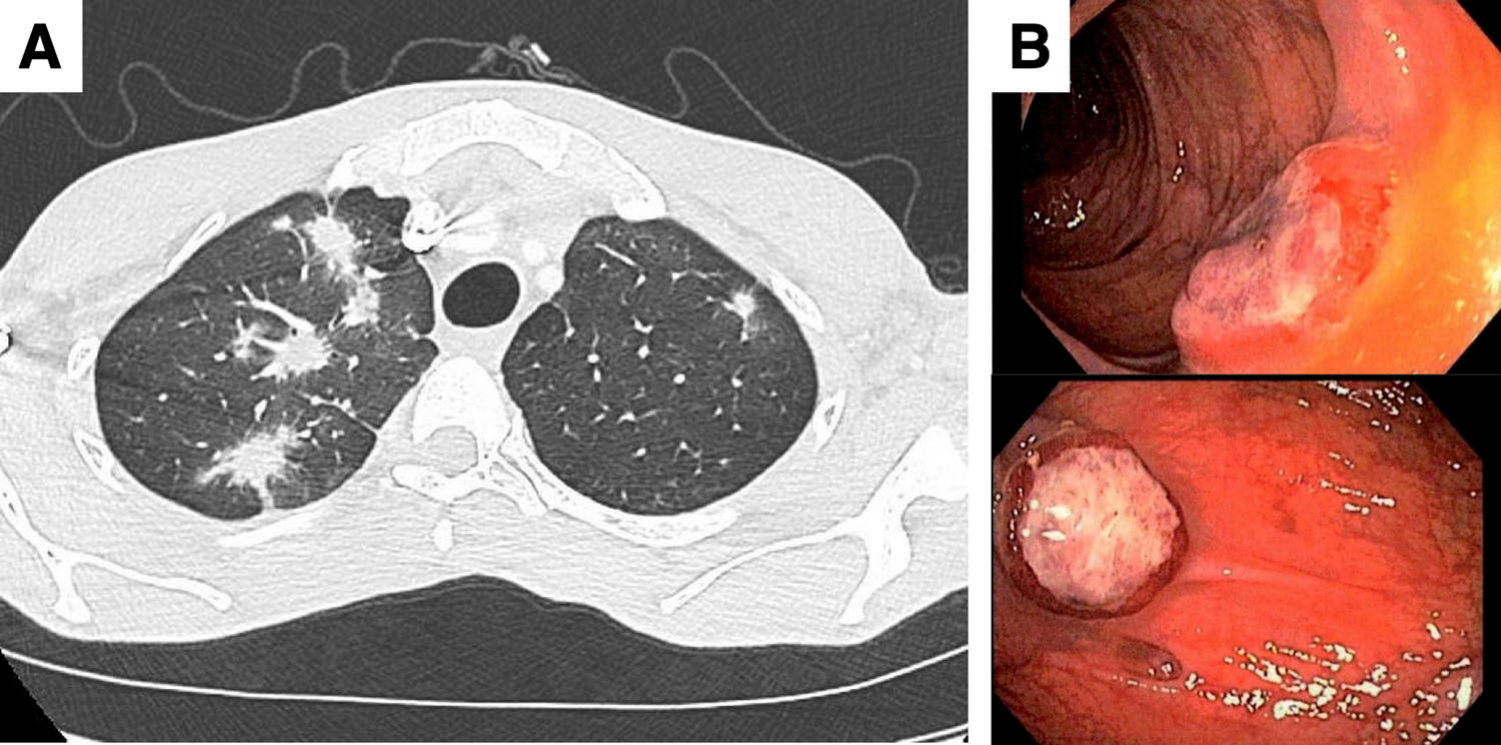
(A) Pulmonary lesion of Kaposi sarcoma identified by chest CT scan, and (B) digestive localizations of Kaposi sarcoma evaluated by colonoscopy in immunodeficient patients with invasive Kaposi sarcoma.
